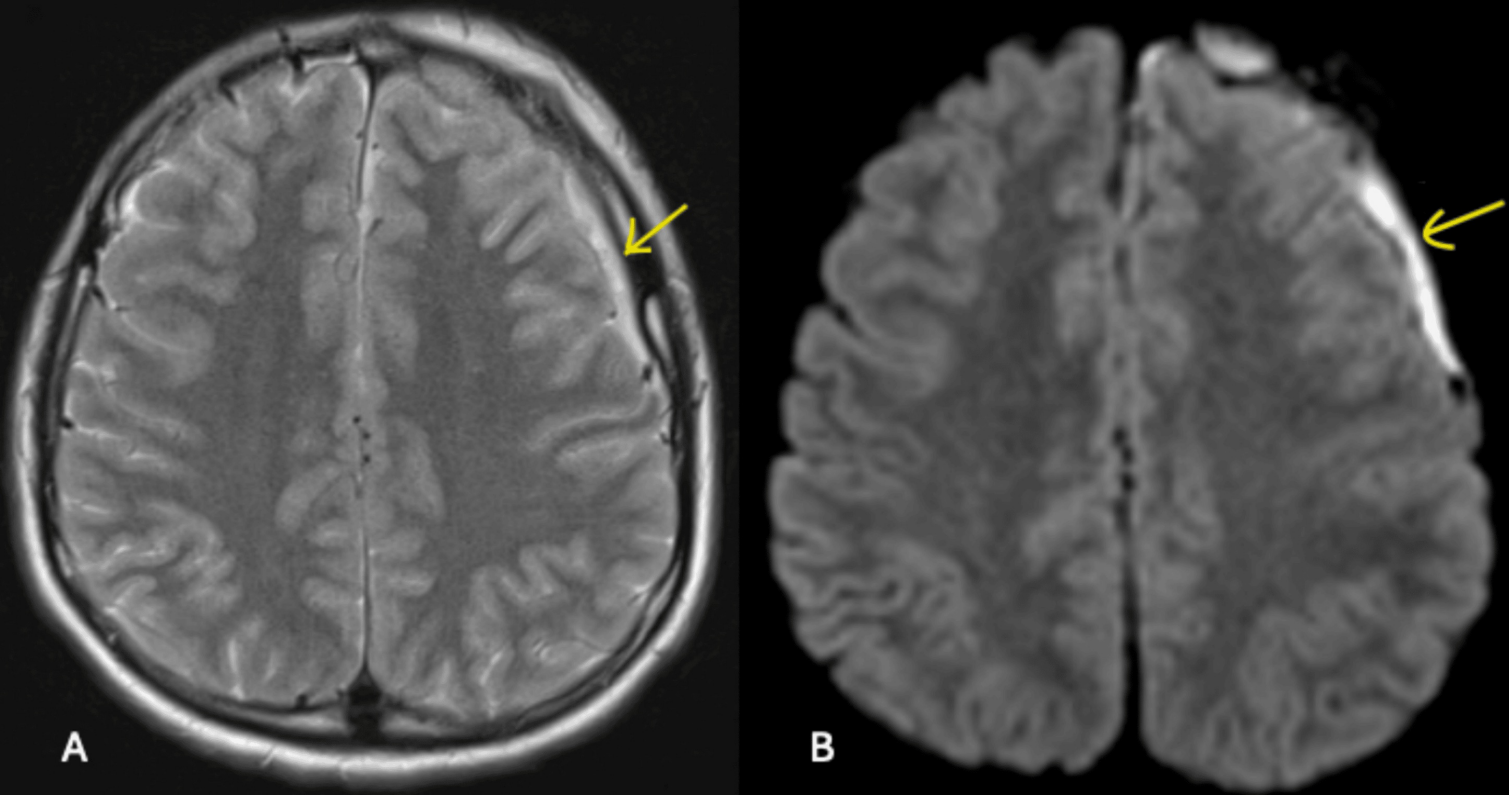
Non-contrast MRI head and sinus in the axial view shows left-sided subdural empyema surrounding the left anterior cerebral convexity in T2-weighted (A) and diffusion-weighted imaging (B) (arrows).
